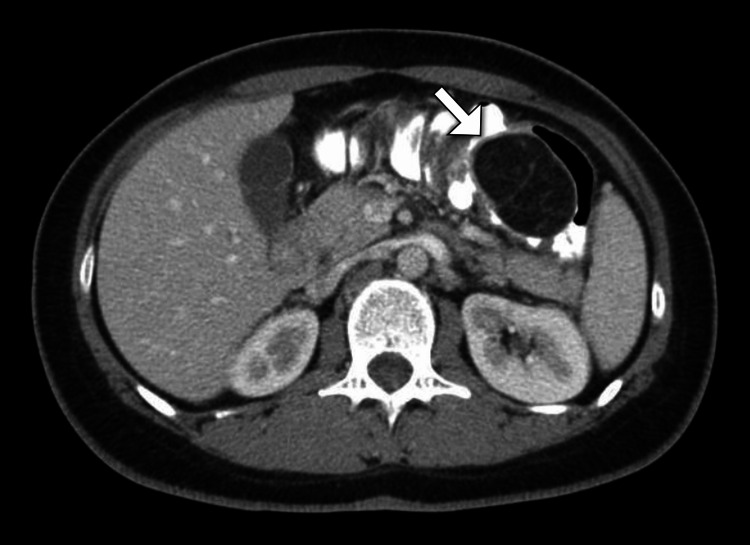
Axial CT scan of the abdomen demonstrates a lesion with fat-density characteristics (arrow) located within the ascending colon CT: computed tomography
